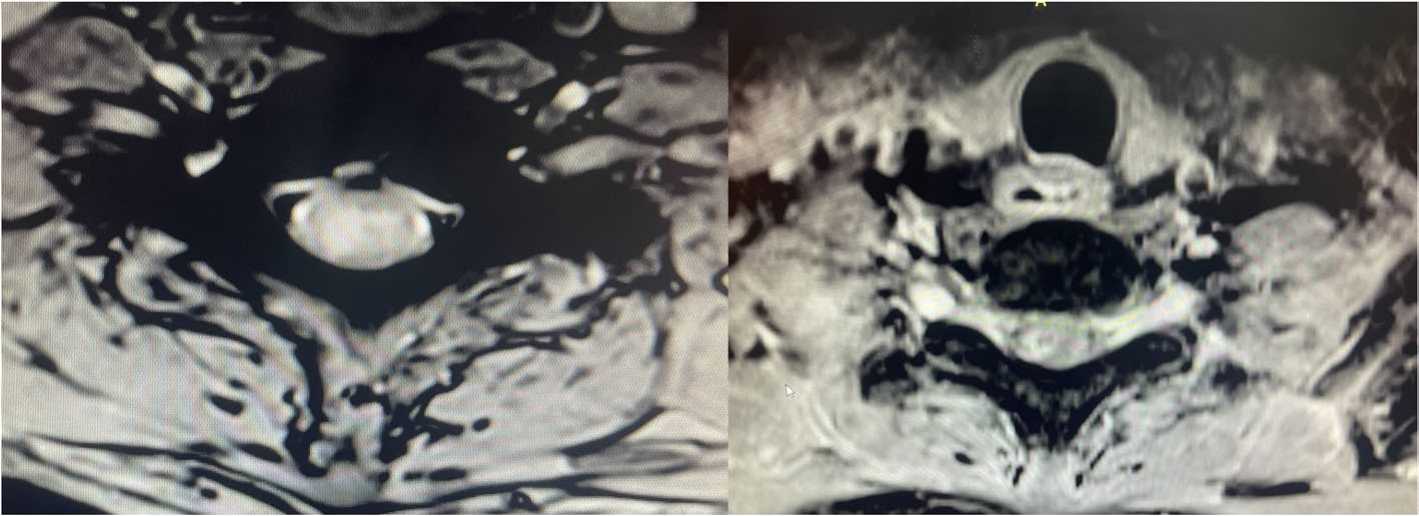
T2W Fat suppressed MRI spine axial image at C6 level showing central hyperintensity and post contrast image showing contrast enhancement
